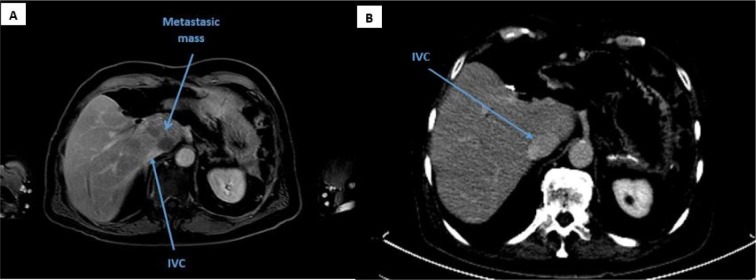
(A): MRI, nine weeks prior to the therapy, revealing the metastatic mass in the liver with an impending IVC compression (IVC diameter: 18.4 x 8.1 mm), (B): CT scan, Twelve months after last PRRT cycle with significant decompression of the vein (IVC diameter: 30.9 x 19.9 mm)
